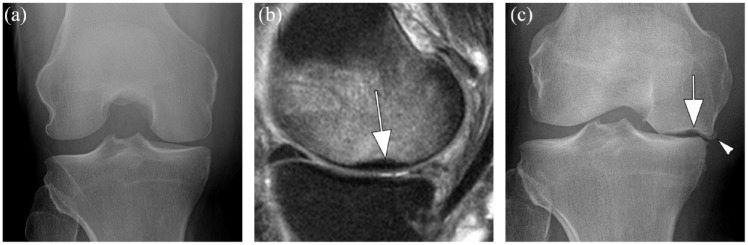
Structurally relevant safety findings may be occult on radiography. (a) anterior-posterior radiograph shows a normal knee joint without surface abnormalities. At the time the patient complained of severe medial knee pain without trauma. (b) Corresponding sagittal proton density-weighted MR image obtained at the same day shows diffuse bone marrow edema in the medial femoral condyle and a focal area of subchondral hypointensity representing subchondral insufficiency fracture (SIF) (arrow). SIF is commonly treated non-surgically including protected weight-bearing. While SIF per se may heal, it may also progress to articular collapse. (c) X-ray obtained 18 months after the initial images were acquired shows an osteochondral defect (arrow), medial joint space narrowing and artciular surface collapse as a result of SIF. In addition there is a small osteophyte that developed at the femoral joint margin (arrohead).
